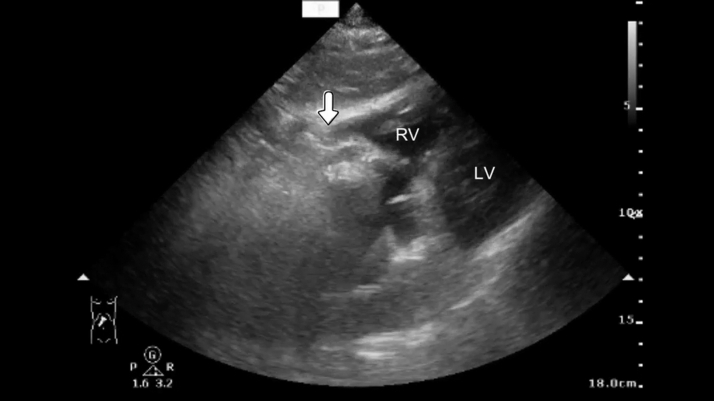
Real-time point-of-care ultrasonography with subcostal four-chamber view and inferior vena cava long-axis view demonstrates multiple hyperechoic foci with posterior dirty shadowing artifacts swirling within the pericardial space, consistent with pneumopericardium. The characteristic movement of air bubbles with cardiac motion is clearly visible.
